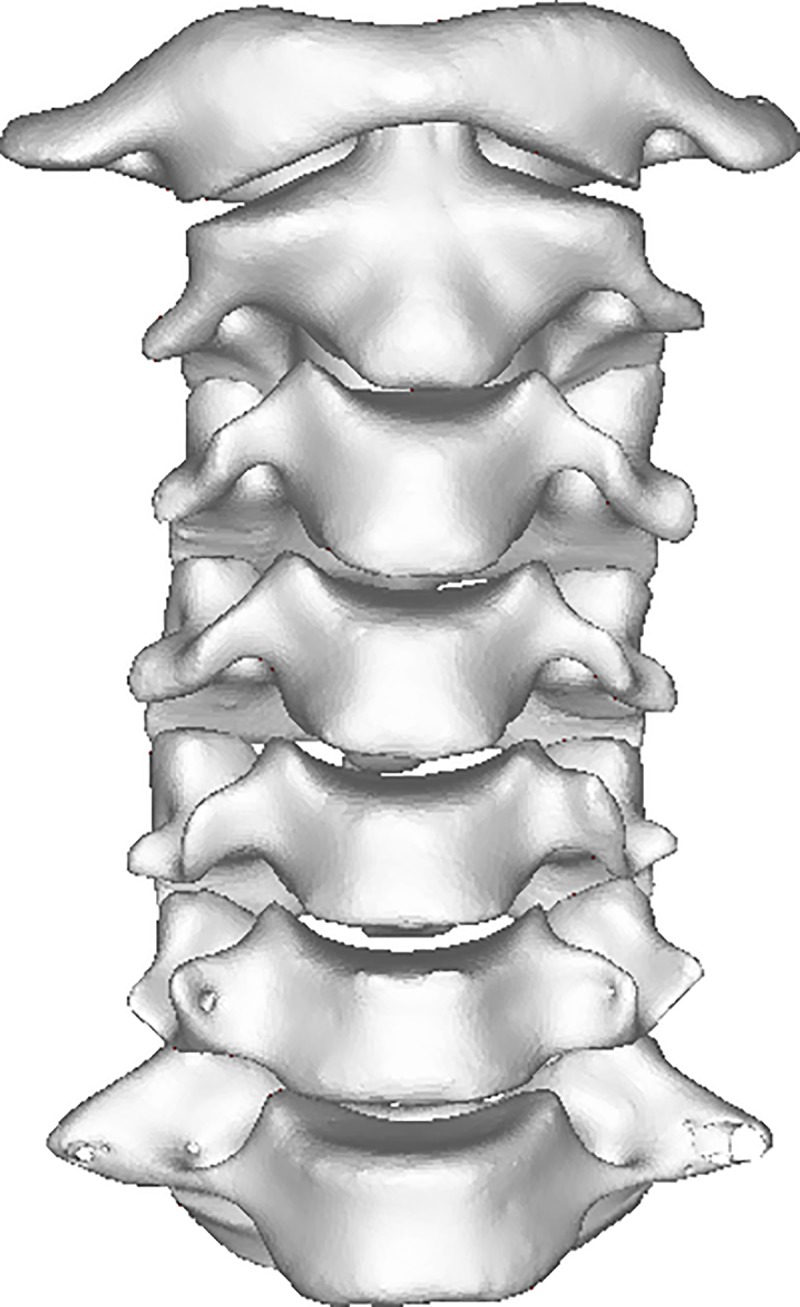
CT 3D reconstruction image of cervical spine: Anterior view.
